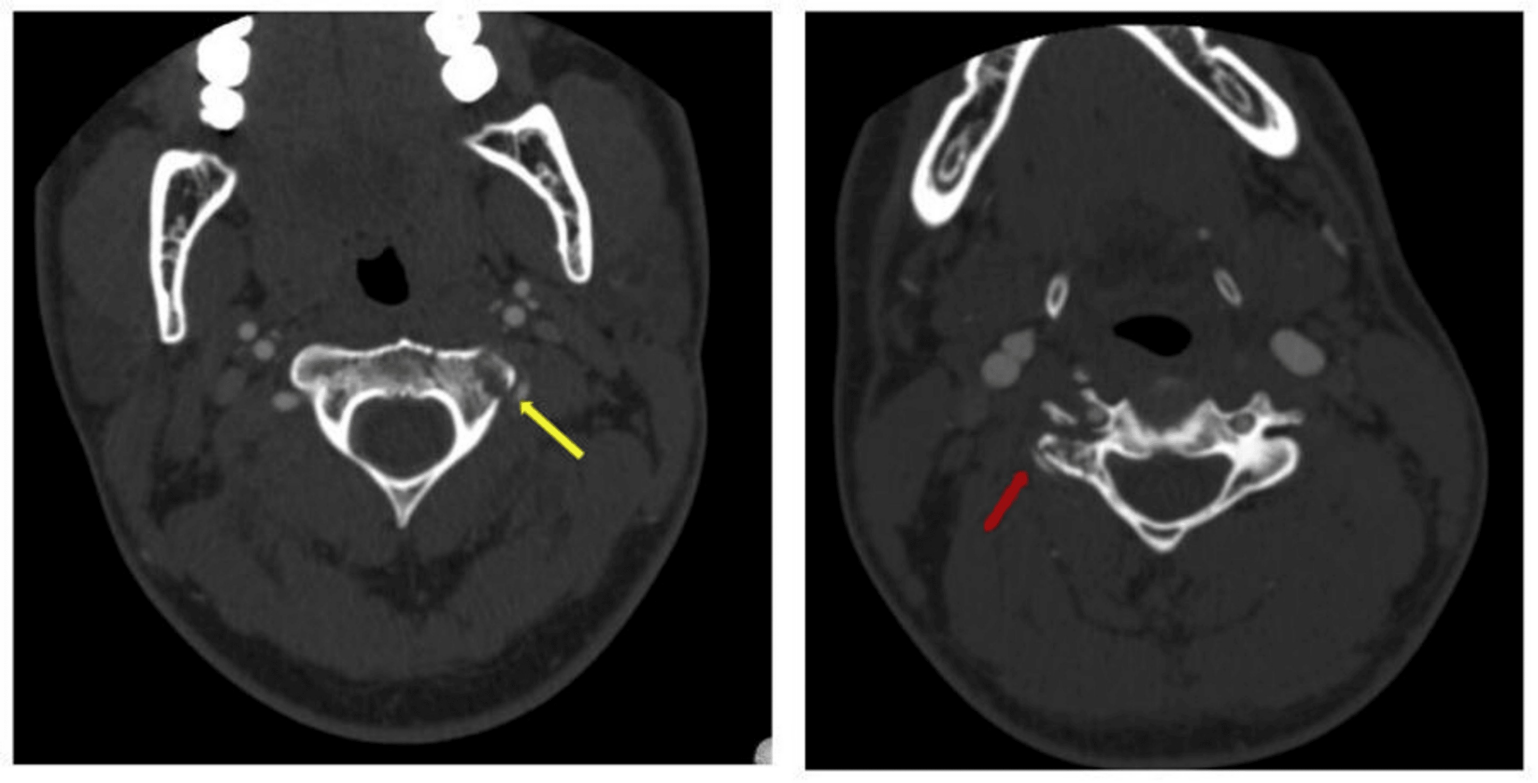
CTA neck axial showing nontraumatic displaced fractures of the left transverse process of C2 (yellow arrow) and right transverse process of C4 (red arrow), concerning for pathological fracture. CTA, computed tomographic angiography.
